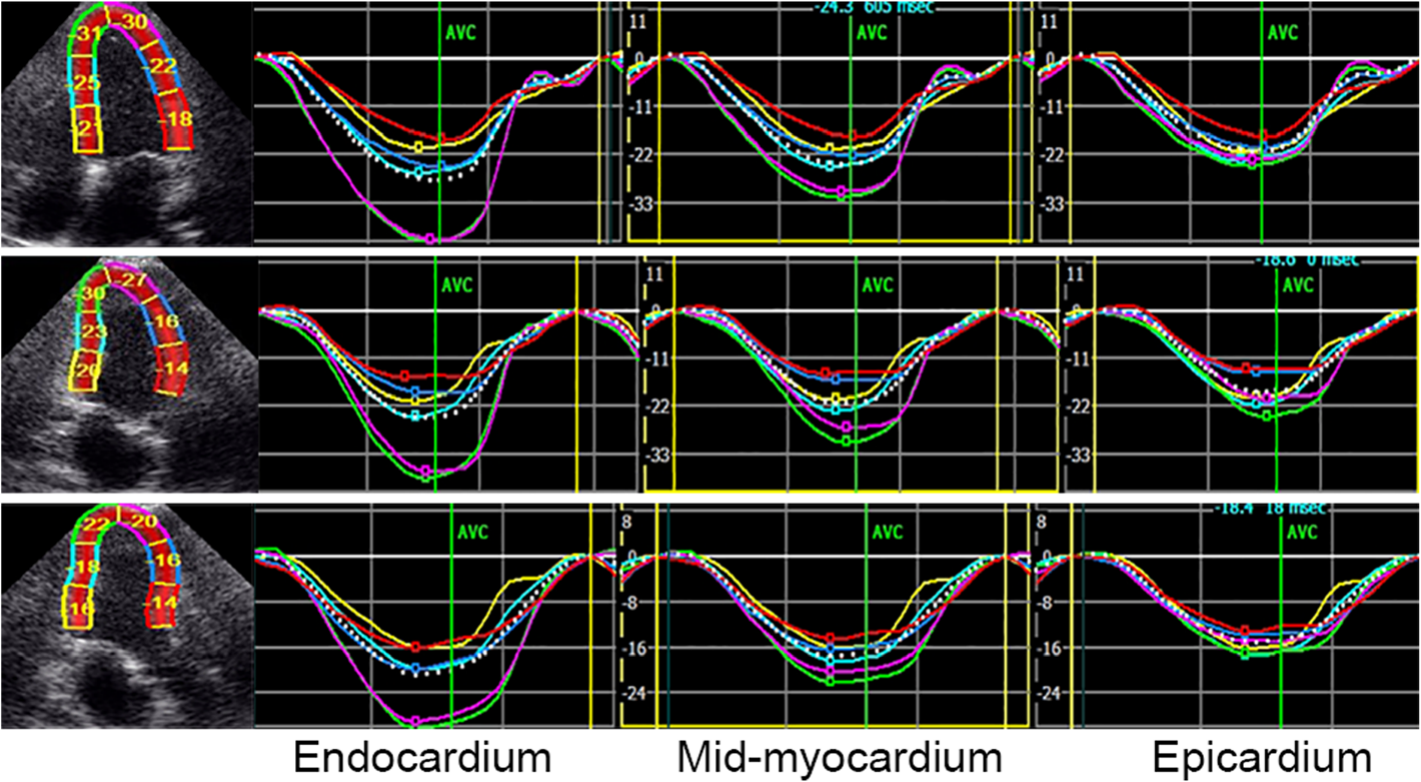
Layer-specific strain curves in each segment. Quantitative myocardial parameters for each segment are evaluated in an 18 segment LV model (six segments at each level) at all three acquired parasternal long-axis views
